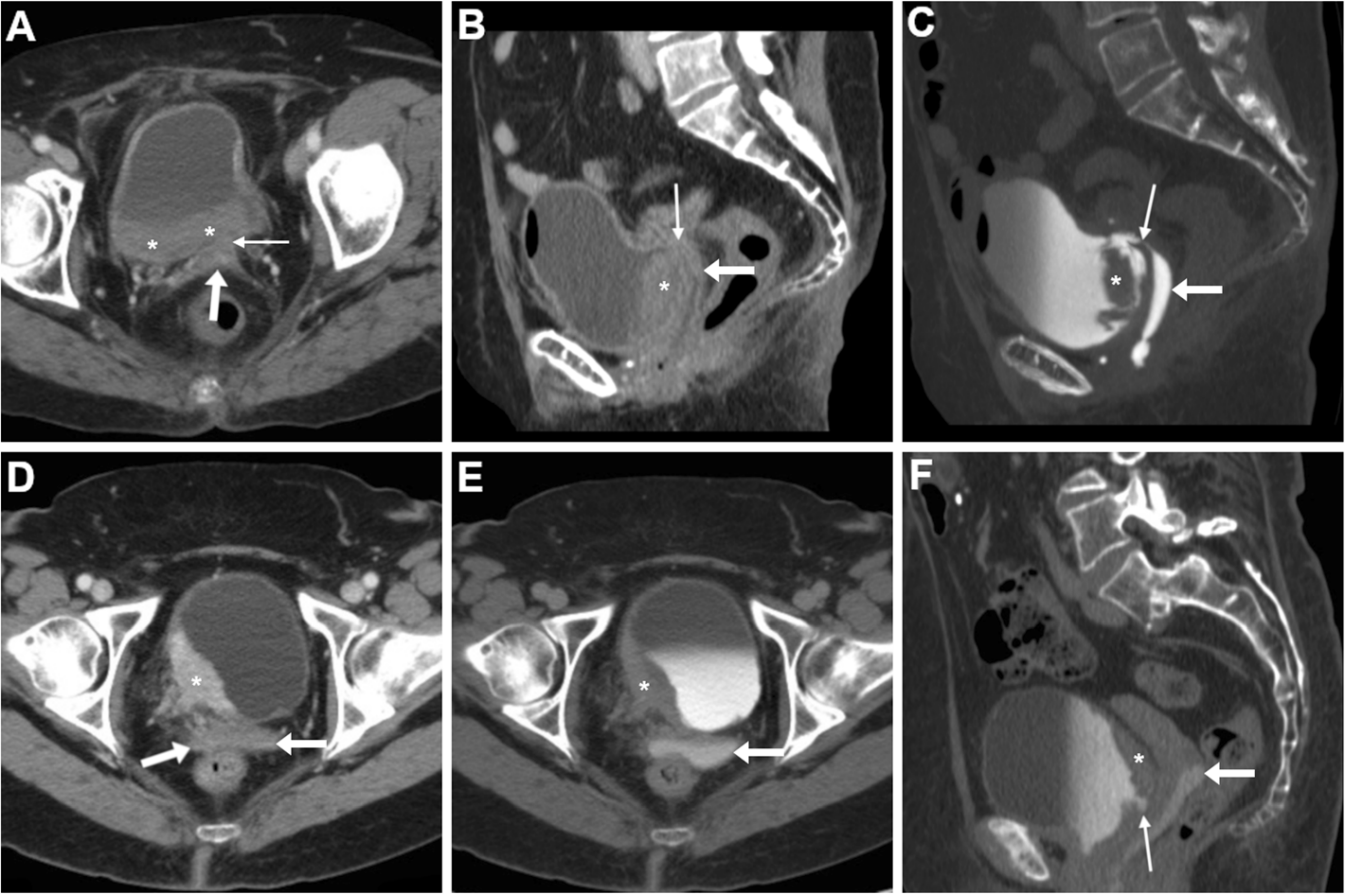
Two cases of urinary VF complicating bladder cancers. a–c In a patient with previous hysterectomy, CT (a, b) shows extensive mural thickening (*) of the posterior bladder aspect, causing adhesion and focal retraction of the bladder dome, and corresponding filling defect on excretory phase acquisition (c). The patent VF (thin arrows) leads to opacification of the vagina (arrows). d–f In a patient with strongly enhancing tumour (*) of the right posterolateral aspect of urinary bladder (d), excretory phase acquisition (e, f) shows vaginal opacification (arrows) via a short VF (thin arrow in f)
